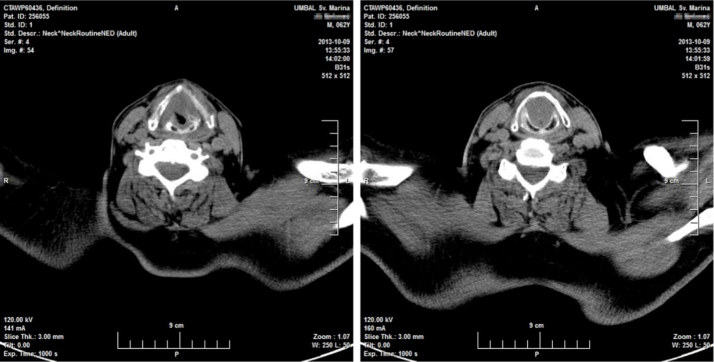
Axial computed tomography (CT) at the level of the thyroid (left) and cricoid (right) cartilages with complete lumen obstruction and no evidence cartilage invasion. The patient breathes through a tracheostomy.
